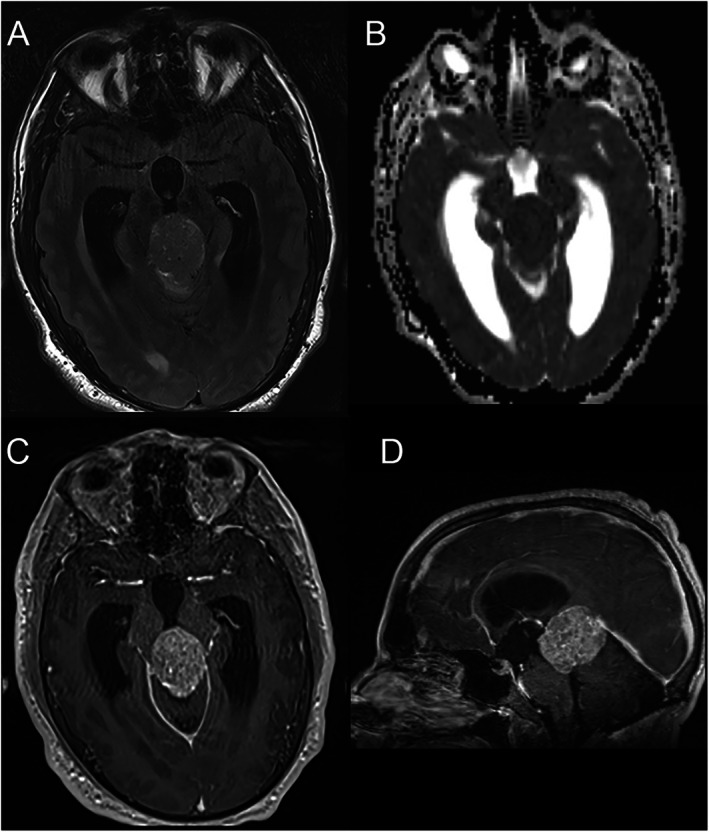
MRI at presentation of the metachronous pineal germinoma. (A) Axial T2‐weighted FLAIR image shows a lobulated extra‐axial, solid mass centered in the pineal region, moderate obstructive hydrocephalus, enlargement of the third and lateral ventricles, and periventricular FLAIR hyperintensity indicating transependymal CSF flow. (B) Axial apparent diffusion coefficient map shows low signal in the mass, consistent with diffusion restriction. (C and D) Axial and sagittal post‐contrast T1‐weighted images show heterogeneous enhancement and mass effect on the tectum and midbrain.
